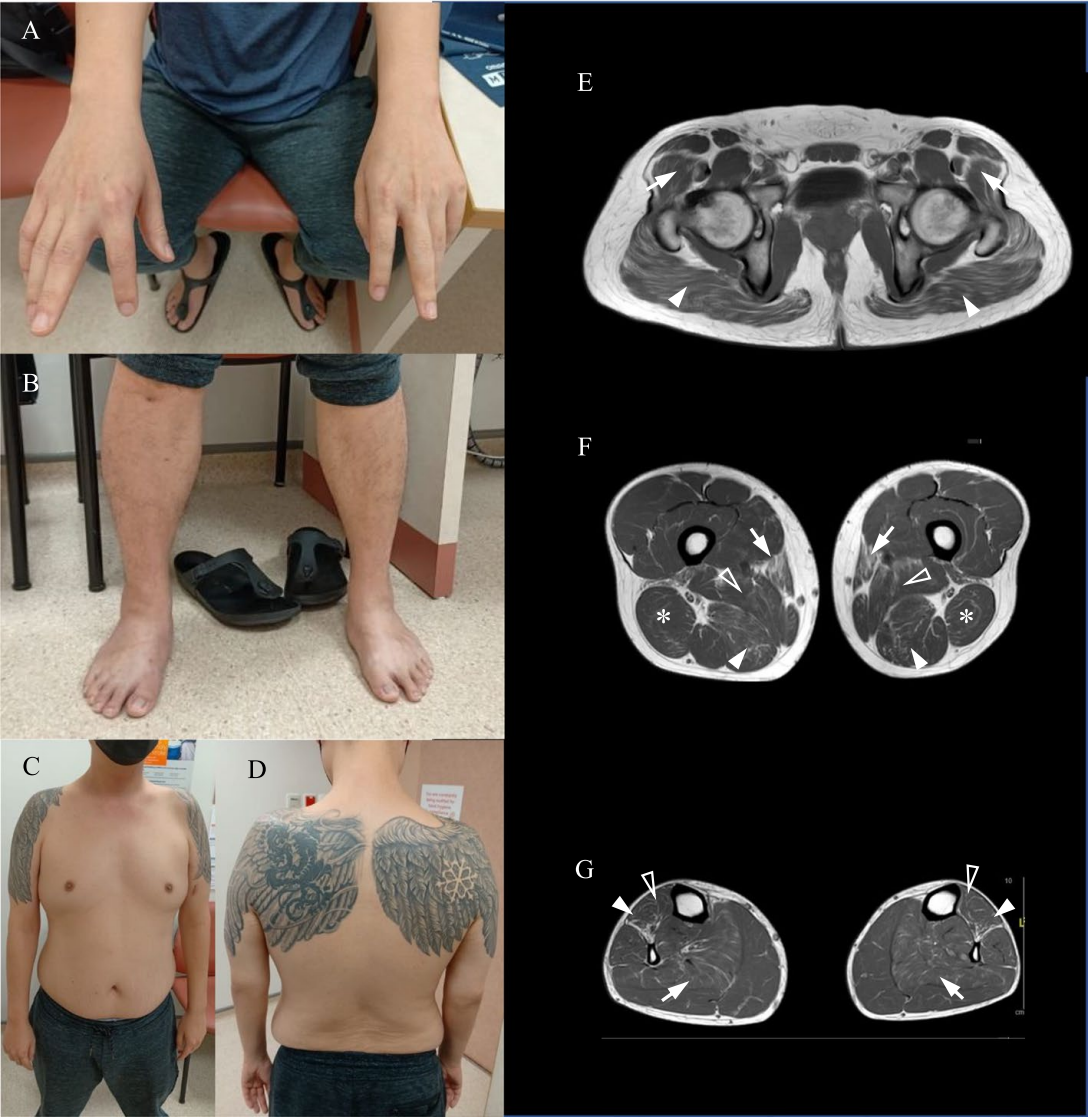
Physical examination findings and lower body MRI of patient. Differential asymmetric distal upper limb weakness of finger extensors and finger abductors (A). No significant atrophy is observed (B-D). Muscle MRI T1 sequence findings of patient at the level of the pelvic girdle (E), thighs (F) and calves (G). There is no significant loss of muscle volume or presence of muscle edema. E Mild symmetric fatty infiltration noted of the bilateral gluteus maximus (arrowhead) and gluteus medius (arrow). F Moderate fatty infiltration of the bilateral sartorius (solid arrowhead), mild infiltration of the adductor magnus (hollow
